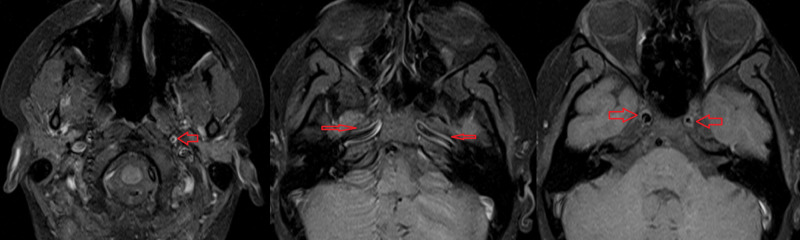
MRI with fat-saturated T1 post-contrast imaging of neck and brain arteries Contrast enhancement on the left distal common carotid artery (right). Active contrast enhancement on the wall of the petrous segment of bilateral internal carotid arteries (middle). Contrast enhancement on bilateral cavernous segments of internal carotid arteries (left).
